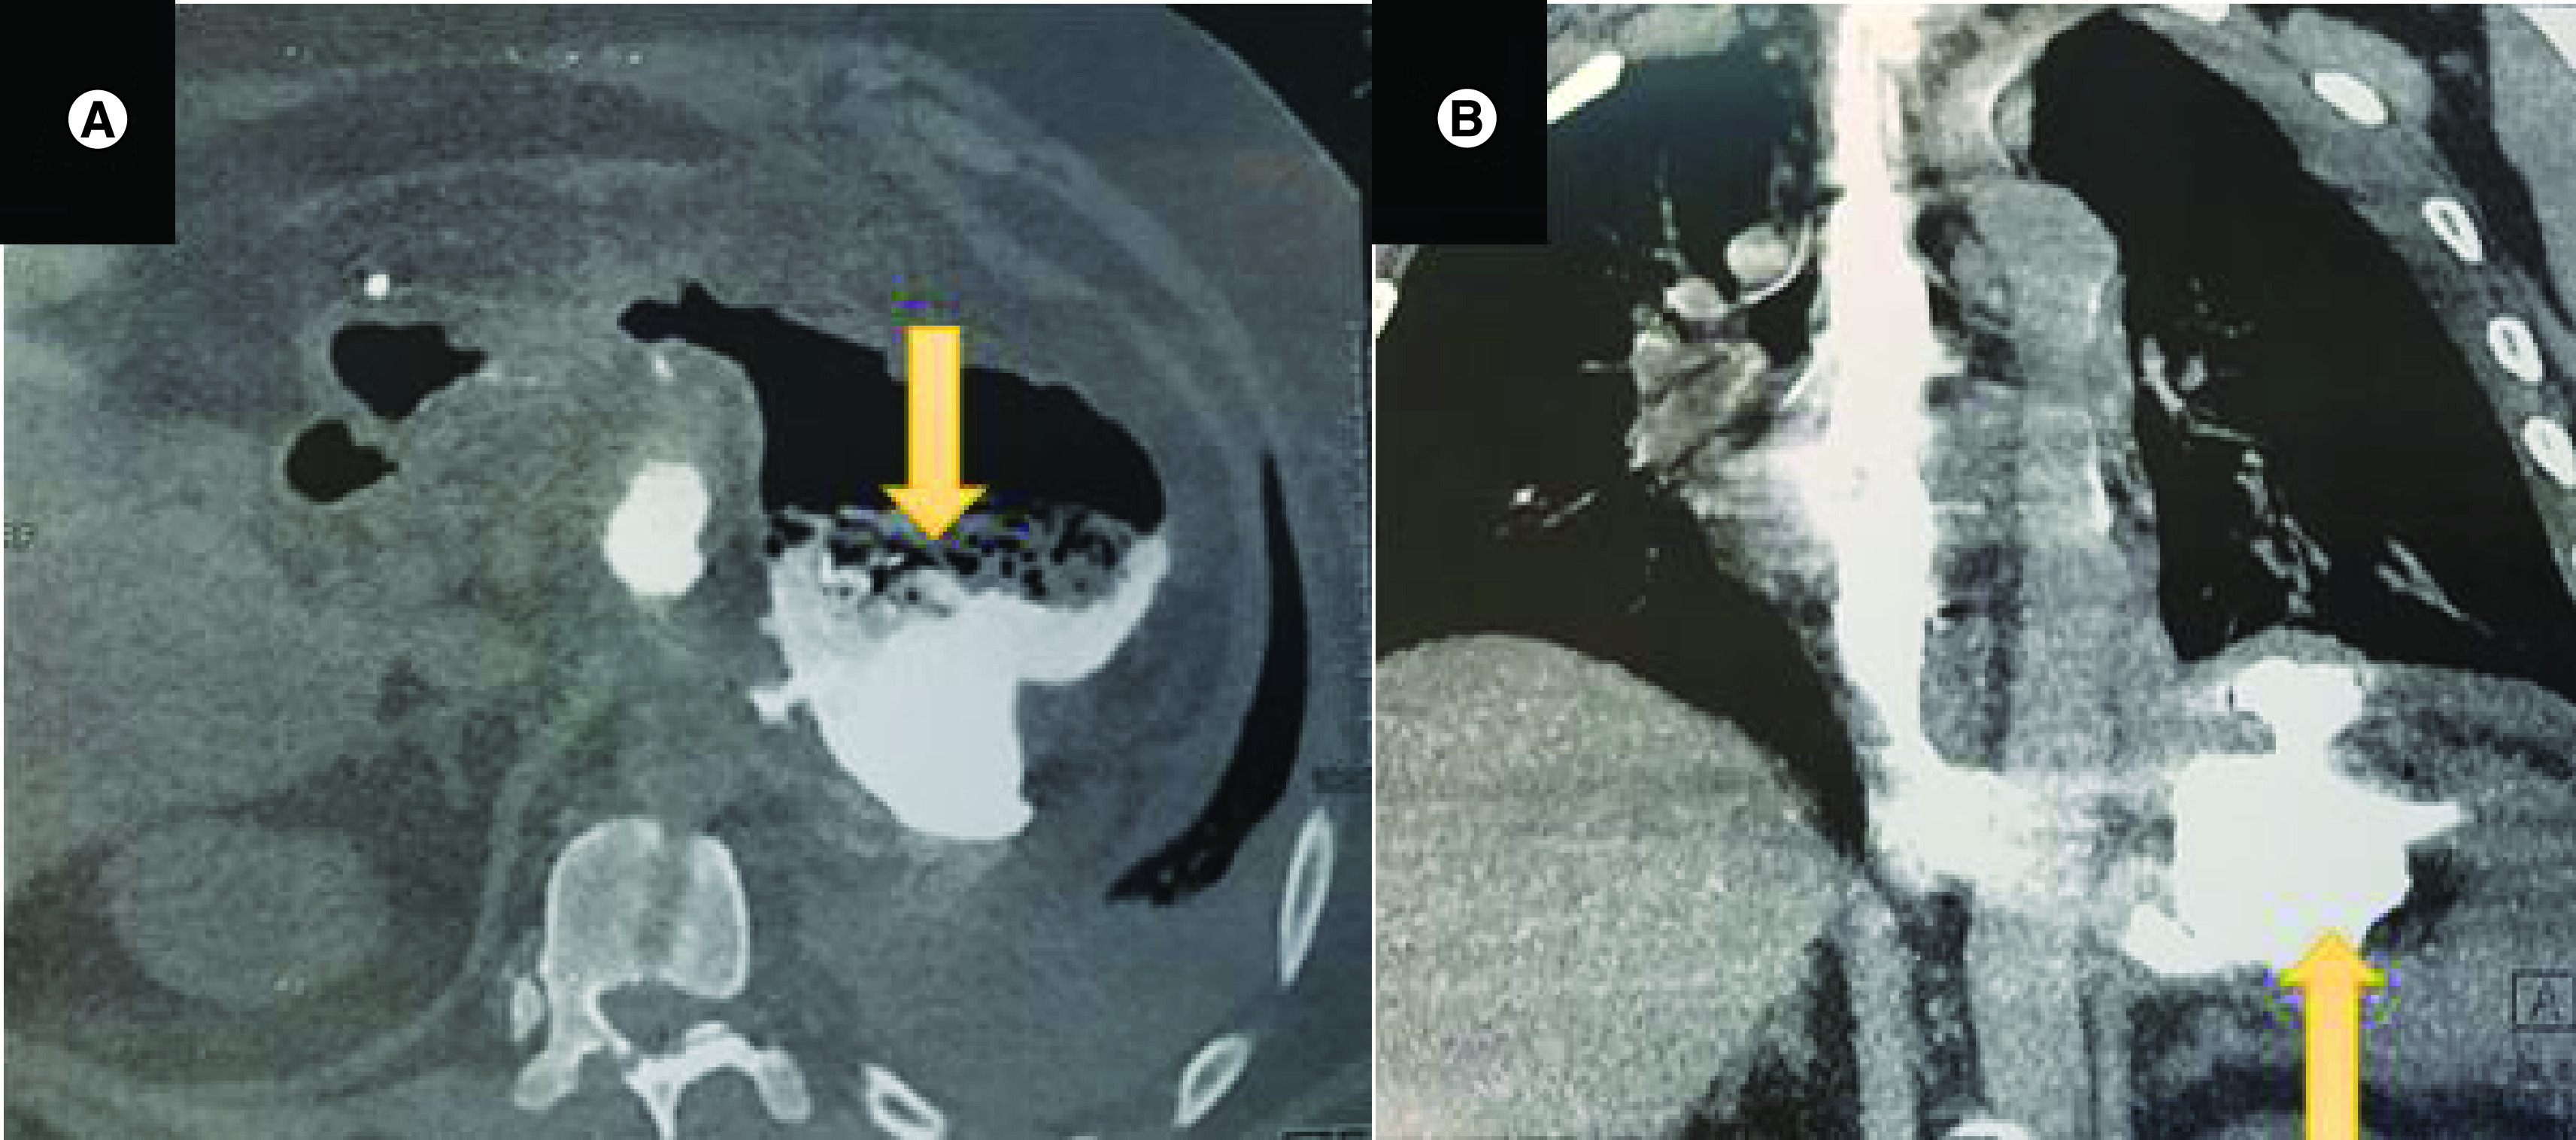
(A) CT scan axial view showing a left subphrenic collection (yellow arrow) communicating with the gastric sleeve. (B) CT scan coronal view showing the left subphrenic collection (yellow arrow).
